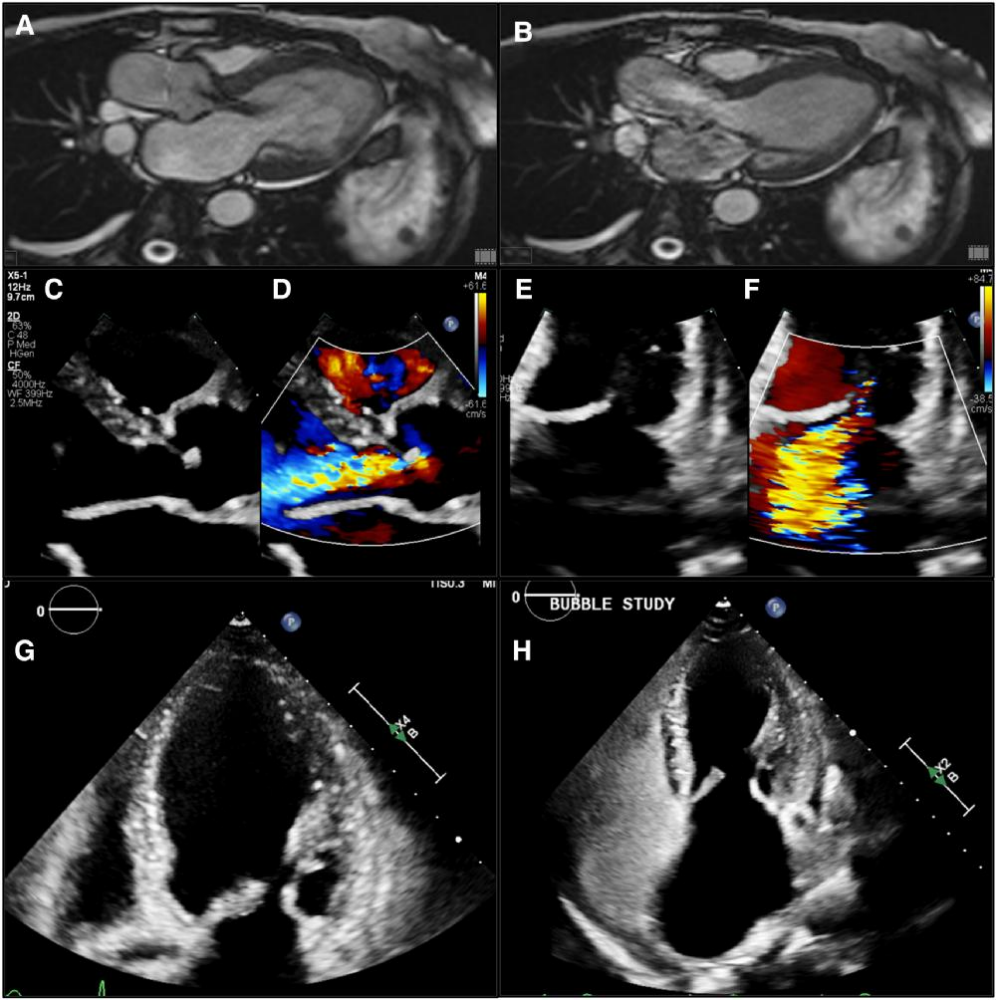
Multimodality imaging findings in a patient with bilateral carcinoid heart disease in the absence of an intracardiac shunt. Panel (*A*) shows a cardiac magnetic resonance three-chamber steady-state free precession cine revealing aortic regurgitation and a thickened anterior mitral valve leaflet, while panel (*B*) demonstrates severe mitral regurgitation on the same view. Panels (*C*) and (*D*) display transthoracic echocardiogram images highlighting the mitral valve morphology with thickened, retracted leaflets and colour Doppler confirming severe mitral regurgitation, respectively. Panel (*E*) shows the transthoracic echocardiogram image of the aortic valve morphology, and panel (*F*) depicts colour Doppler evidence of moderate aortic insufficiency. The apical four-chamber view in panel (*G*) further demonstrates thickened mitral valve leaflets. Finally, panel (*H*) presents an agitated saline contrast study confirming the absence of a right-to-left shunt.
